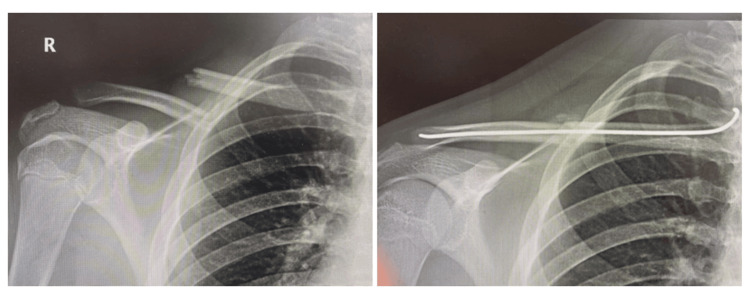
Comparison of X-rays between the initial clavicle fracture (left) and the post-operative placement of the intramedullary nail, showing 100% radiological union (right) Radiograph clavicle of Patient 1
